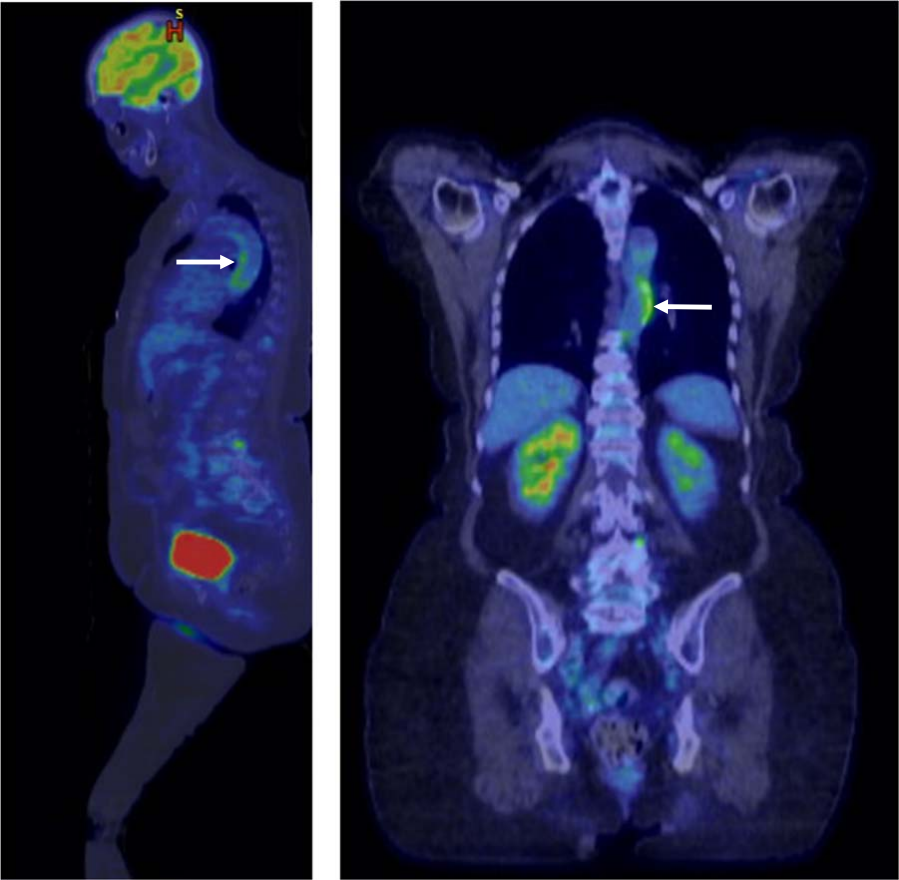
Fluorodeoxyglucose (FDG) PET-CT imaging with mild to moderate arterial FDG uptake most prominent in the descending aorta (white arrows). Based on clinical presentation, laboratory findings, and PET data, the patient met the 2022 American College of Rheumatology criteria for giant cell arteritis.^13^
